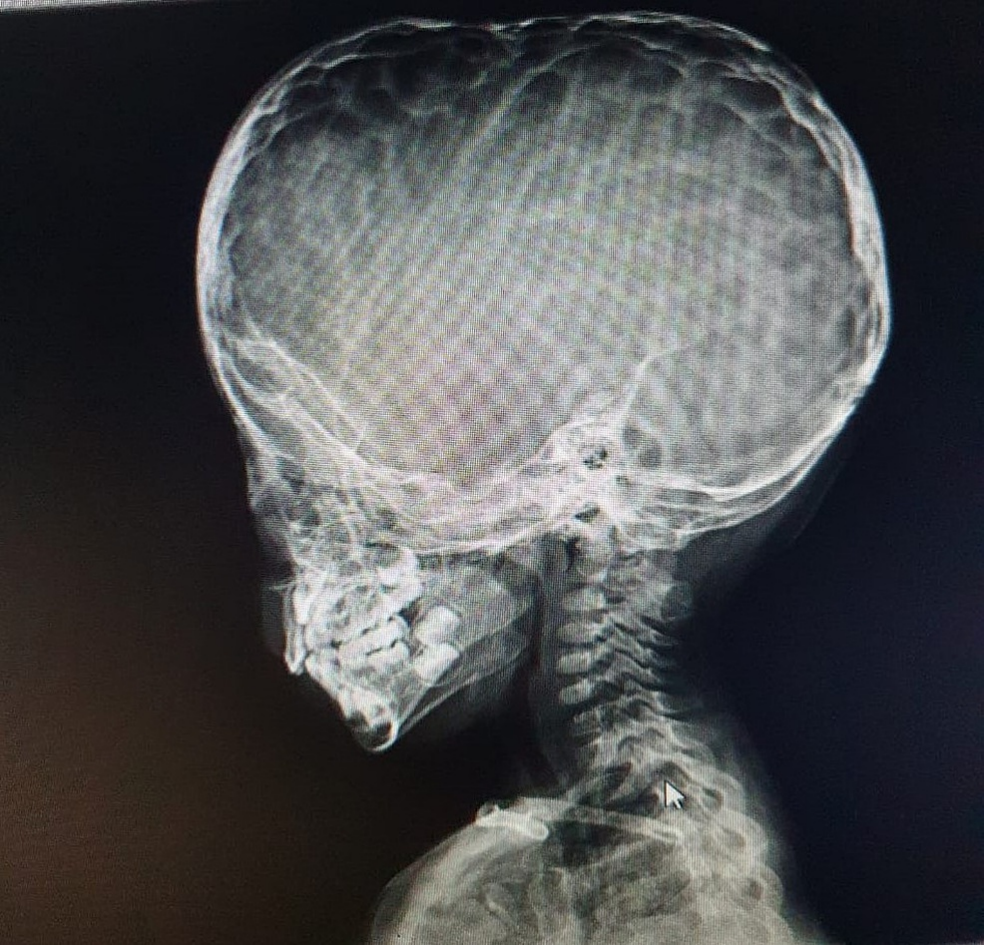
Skull radiography lateral view showing copper beaten appearance with widened roof of sella.
